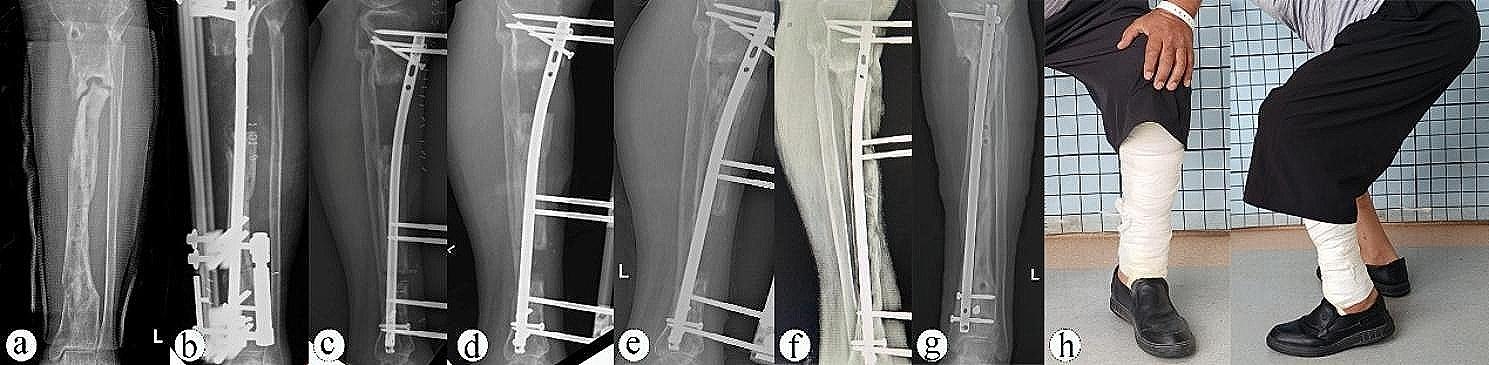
53-year-old male with a left tibial bone defect caused by post-traumatic osteomyelitis was treated by bone transport using EFOIN. a) X-ray showed a nonunion of the left tibia before reconstructive surgery. b, c, d) After radical debridement, the bone defect was approximately 7.3 cm and treated by bone transport using a unilateral external fixator over an intramedullary nail. e) Callus was observed in the distraction area without axial deviation at 7 postoperative weeks. f) The transport bone segment reached the docking site at 11 postoperative weeks. g, h) Satisfactory bone healing and function recovery of the left lower limb was achieved at 9 postoperative months
